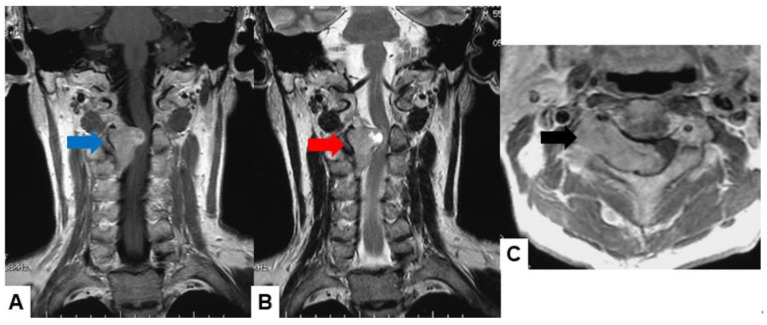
Fifty-five M, spinal schwannoma. (A) Enhanced T1-weighted midsagittal image, (B) T2-weighted midsagittal image, and (C) enhanced T1-weighted axial image at C2/3. Red arrow indicates tumor and blue arrow shows tumor enhancement. The tumor is dumbbell-shaped (black arrow).
